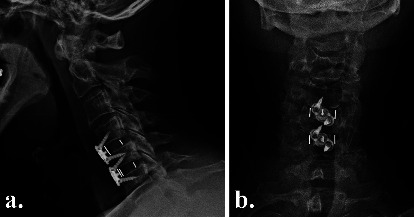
Postoperative (a) lateral and (b) anteroposterior radiographs on day 2, showing NMRT cages with integrated plate/screws at C4/5 and C5/6.
